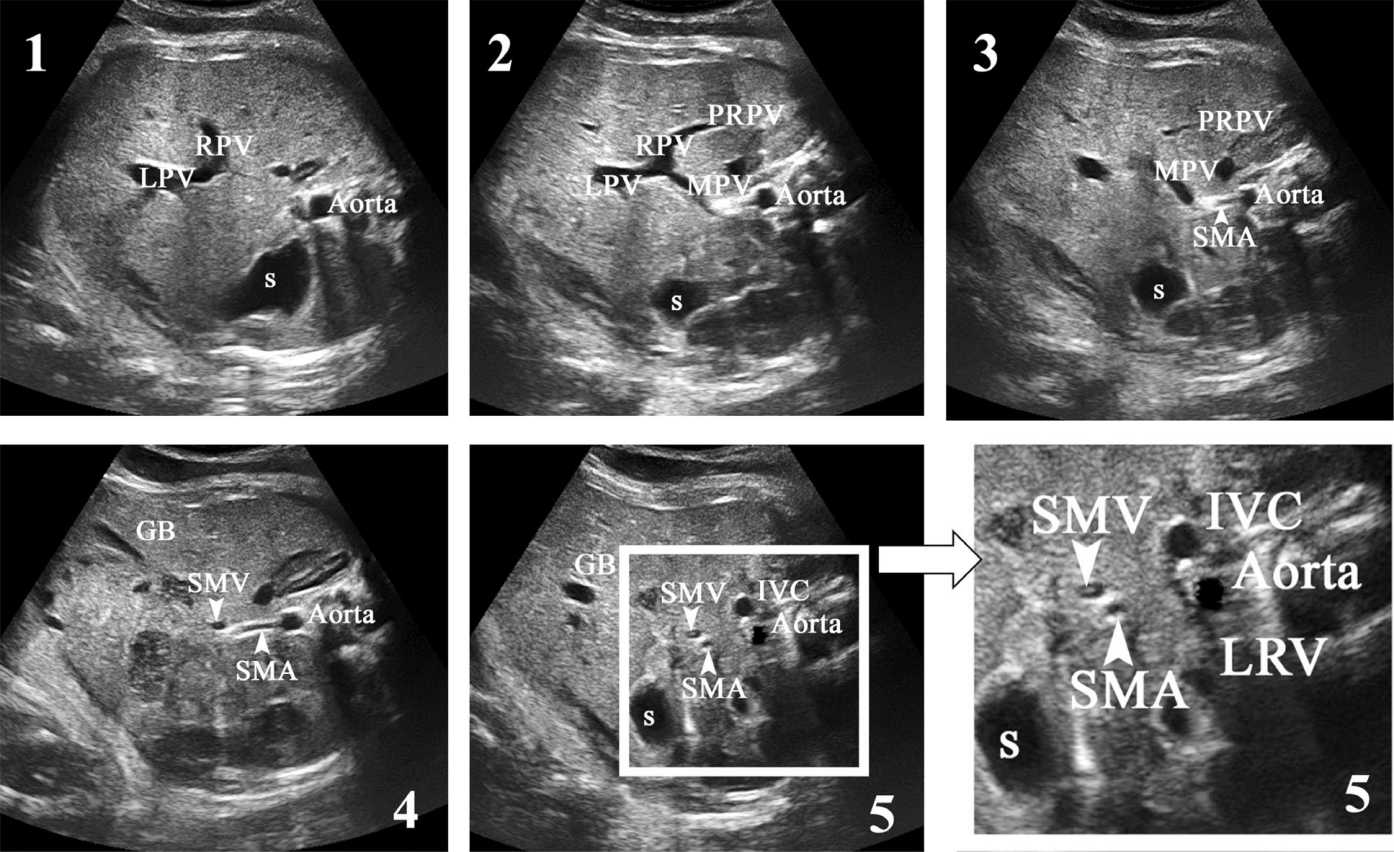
Prenatal ultrasound imaging of the fetal abdomen (transversal view). The left side of the fetus corresponds to the bottom of the image. The portal sinus, corresponding to the junction of the left portal vein (LPV) and the right portal vein (RPV), is visible on the cross‐section of the abdominal circumference (1). The main portal vein (MPV) (2 and 3) arise from the portal sinus with an oblique left and inferior direction and continues with the superior mesenteric vein (SMV) (4). The superior mesenteric artery (SMA) has a peripheral echogenic halo. It originates from the anterior part of the aorta and goes anteriorly and downwards (4). On the reference ultrasound plane (5), the two mesenteric vessels travel in close proximity to each other and are visualized in a strict cross‐sectional plane. Ideally, between the aorta and the SMA the left renal vein (LRV) draining into the inferior vena cava (IVC) is visualized. Posterior branch of right portal vein (PRPV). S, stomach; GB, gallbladder.
